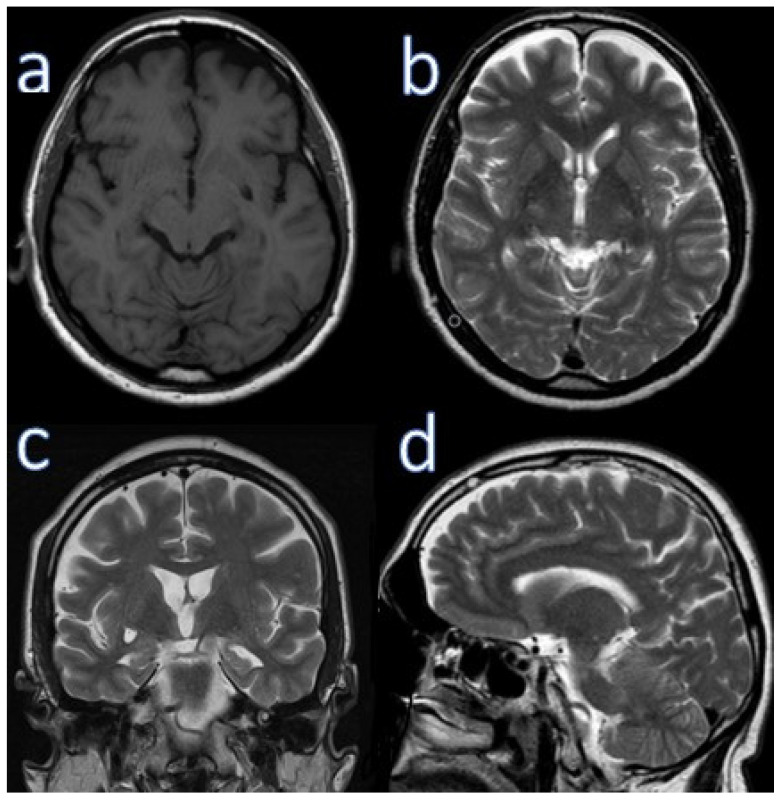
Brain MRI of case 2. (a) Axial T1 weighted, (b) axial T2 weighted (upper row). (c) Coronal T2 weighted, (d) sagittal T2 weighted (lower row).
